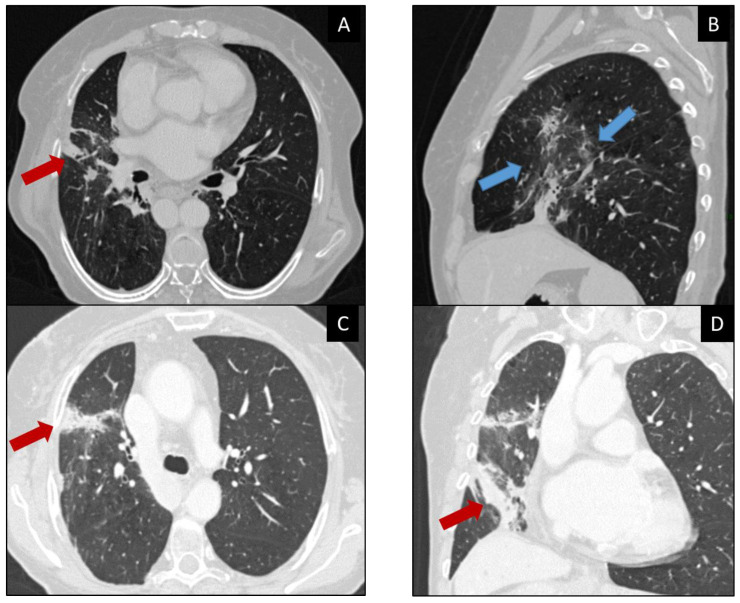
65-year-old patient with history of lung adenocarcinoma (not shown in the images) under Nivolumab. Axial, sagittal, and coronal CT images of the chest (A–D) showing mixed abnormalities of the right lung with consolidations (red arrows) and diffuse ground-glass opacities (blue arrows). ICI-related pneumonitis was confirmed by clinical and laboratory data. Corticosteroids were administered and Nivolumab therapy was suspended with improvement of the clinical picture.
